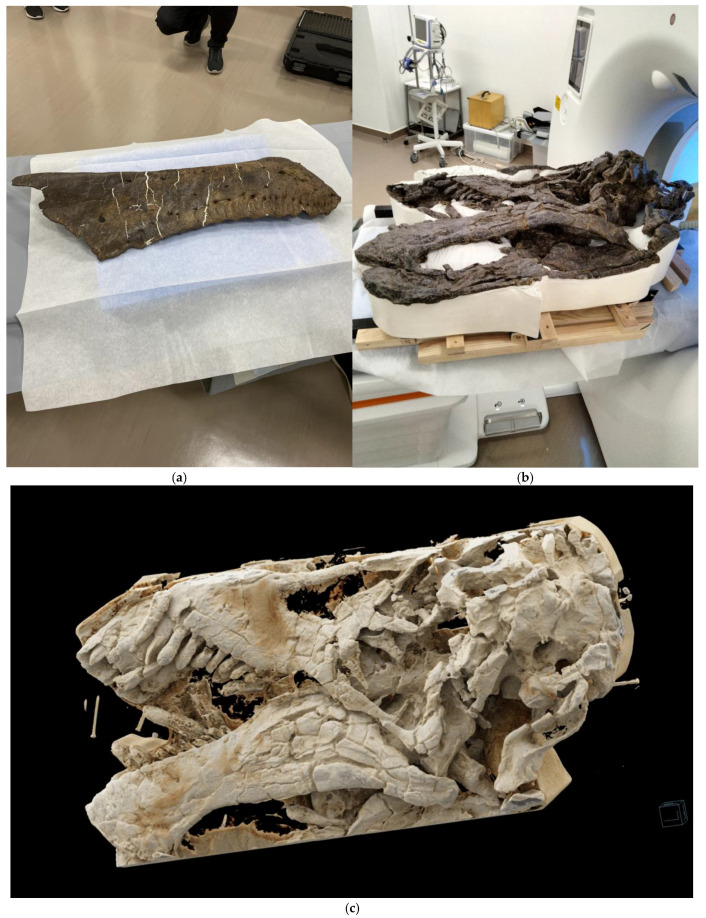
A part of the left dentary of *Tyrannosaurus* (a) and the entire skull of *Camarasaurus* (b) placed on the PCD-CT bed; a 3D image of the entire skull of *Camarasaurus* taken with PCD-CT (c).
